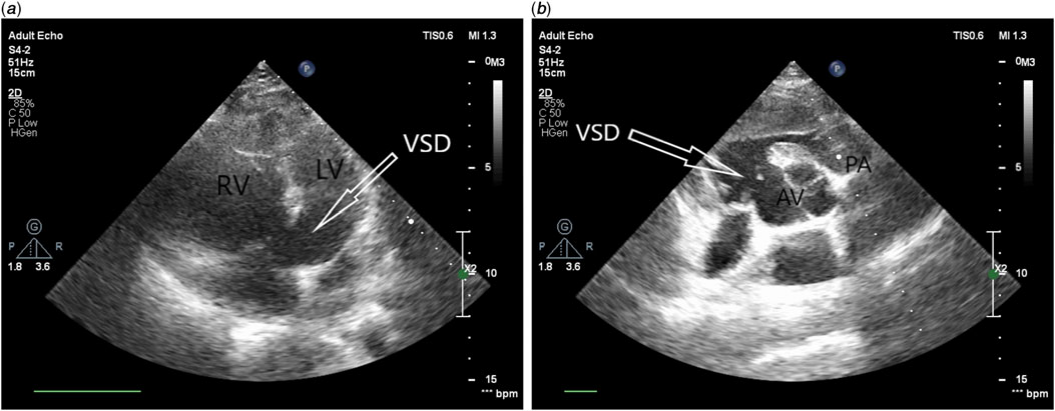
Doppler echocardiography: (*a*) in 4C view shows large sub-aortic VSD with extension to inlet; (*b*) in SAX shows large VSD; VSD: ventricular septal defect; PA: pulmonary artery; AV: aortic valve.
